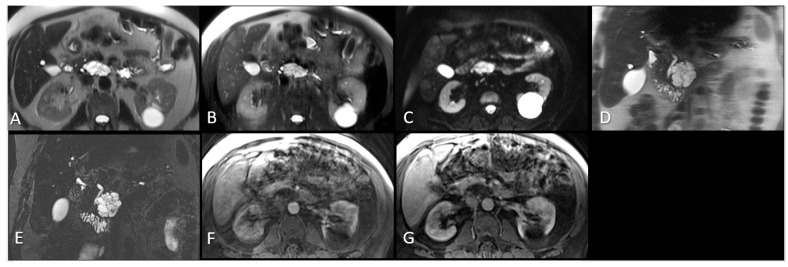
MRI sequences evaluated in this study. (A). Axial T2W HASTE. (B). Axial T2W fat saturated. (C). Axial DWI (low b = 50). (D). Coronal T2W HASTE. (E). Coronal MRCP. (F). Axial T1W fat saturated post contrast arterial phase, (G). Axial T1W fat saturated post contrast venous phase. The maximum diameter was measured on the coronal MRCP series for this uncinate process pathology proven pancreatic IPMN. MRI: Magnetic resonance imaging. W: Weighted. HASTE: Half-Fourier single-shot turbo spin-echo. DWI: Diffusion weighted imaging. IPMN: Intraductal papillary mucinous neoplasm. MRCP: Magnetic resonance cholangiopancreatography.
